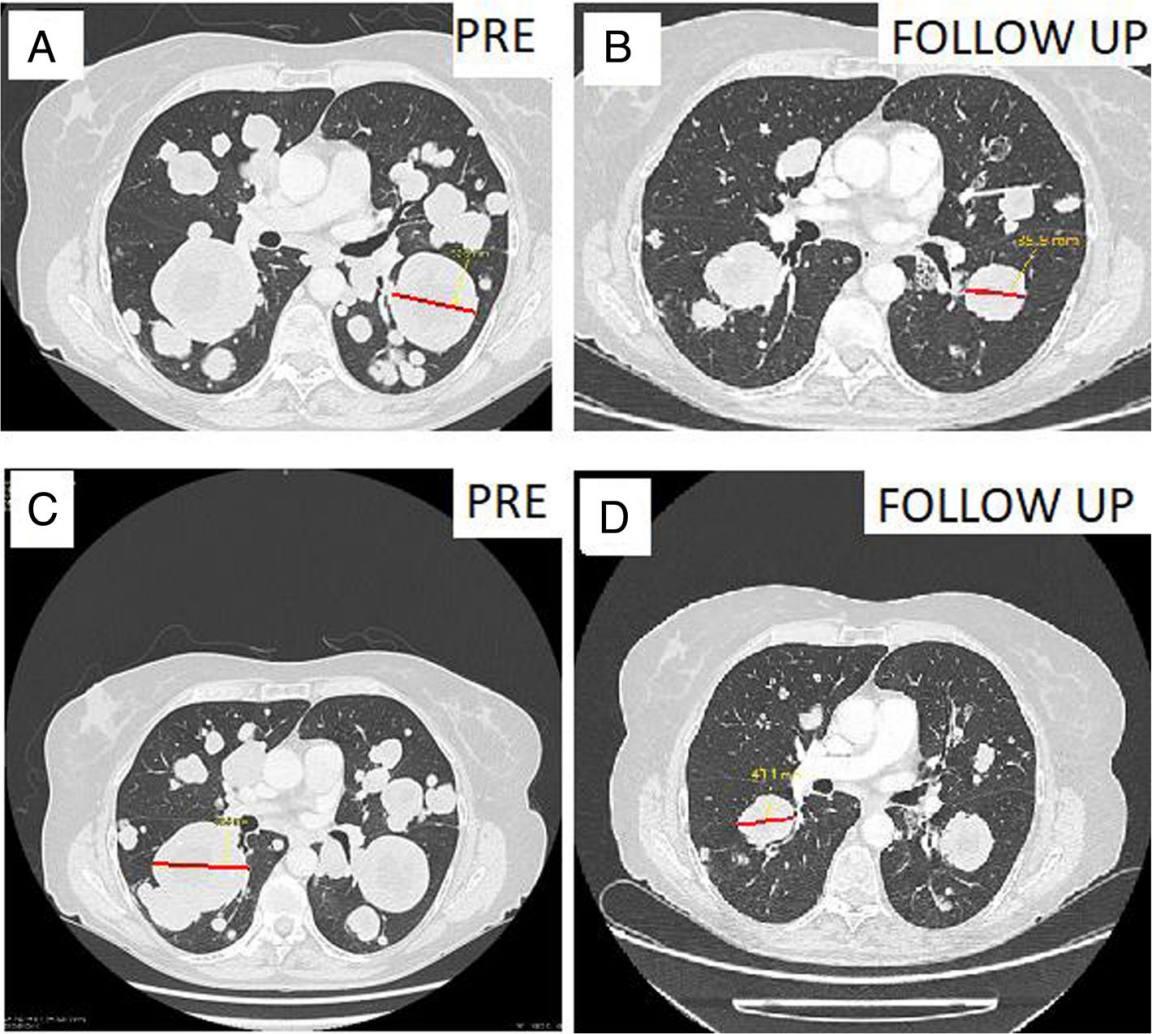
Computed tomographic scan showing response with BRAF inhibitor in a patient with stage IV ameloblastoma. Target lesions were measured before treatment (6 December 2016) and 26 months after treatment (7 February 2018), respectively, at 56 mm (a) and 36 mm (b) in the left apical lower lobe and 64 mm (c) and 43 mm (d) in the right apical lower lobe. Reduction in tumor mass is shown. The red lines denotes the length of lesions taken into account respectively 56 mm for a, 36 mm for b, 64 mm for c and 43 mm for d
